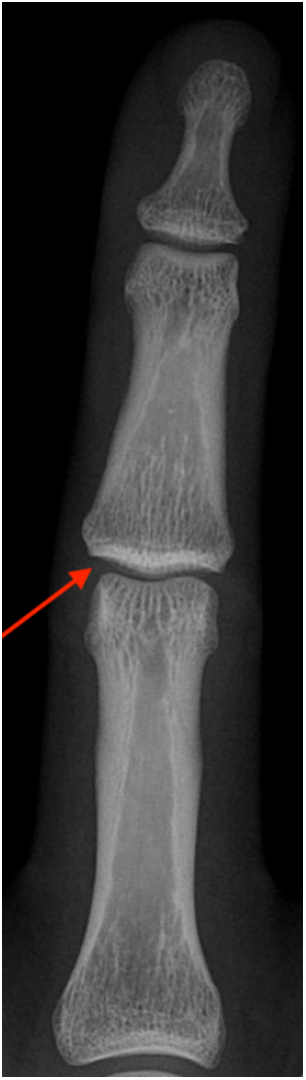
X-ray of a capsulitis of the PIP joint depicting as a joint space widening due to the effusion (“x-ray sign 1”, arrow) as well as a thickened soft tissue presentation (“x-ray sign 2”) (9).
